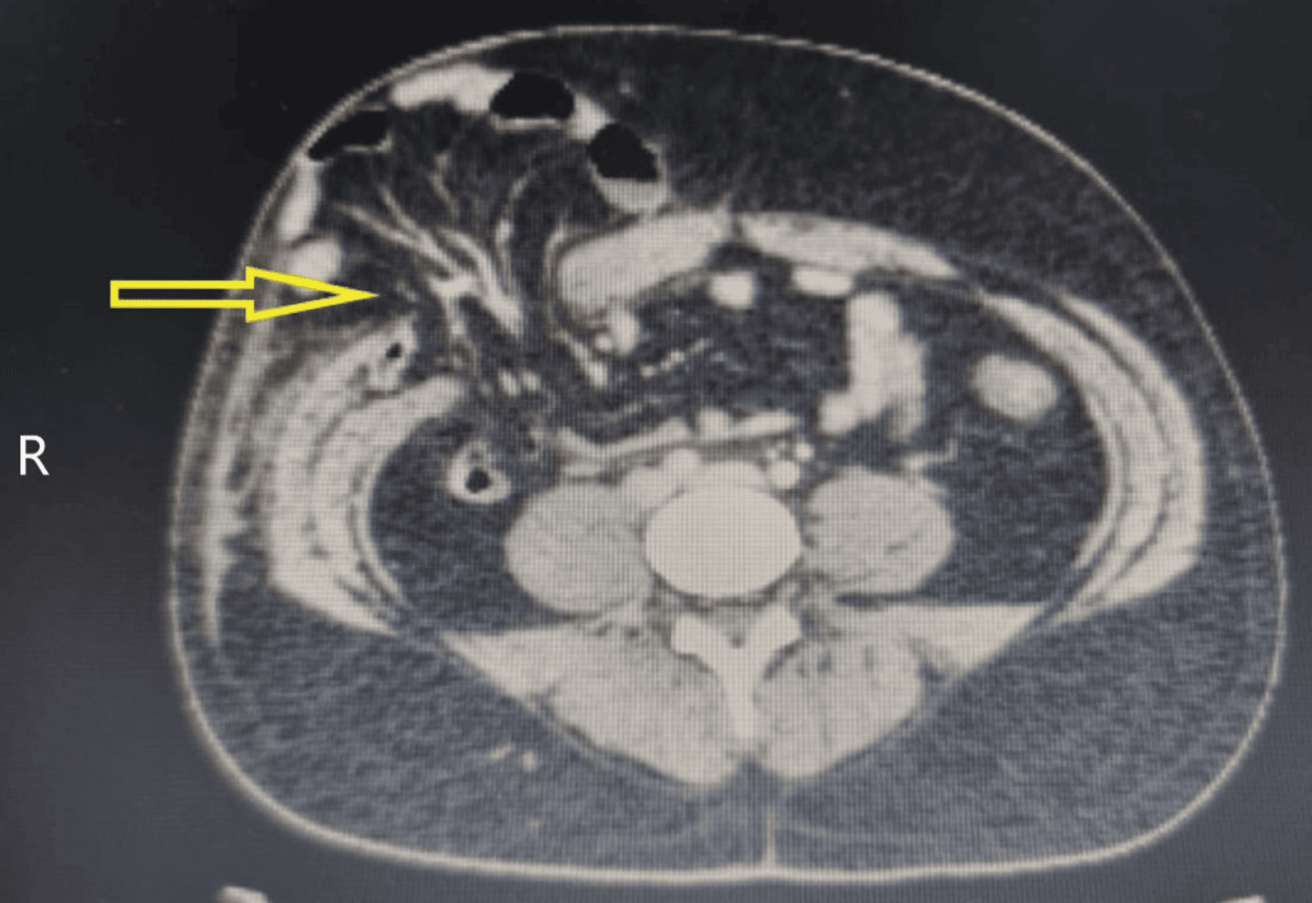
CT scan of the abdomen shows the hernia defect and the protruding contents of the hernia through the defect. CT: computed tomography
